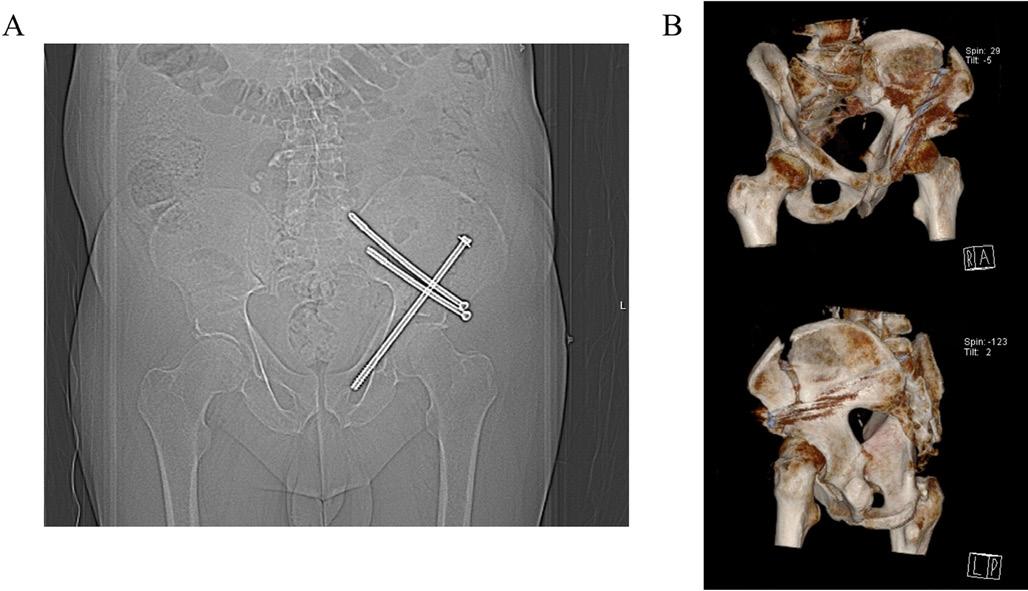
Closed reduction and screw fixation of left acetabular fracture. (A) Plain film radiograph demonstrating a left-sided long lag screw, with distal end lying within the superior pubic ramus. Further screws were placed through the supra-acetabular ilium. (B) Three-dimensional reconstruction of CT of the pelvis demonstrating a long lag screw and two supra-acetabular screws, as described above.
